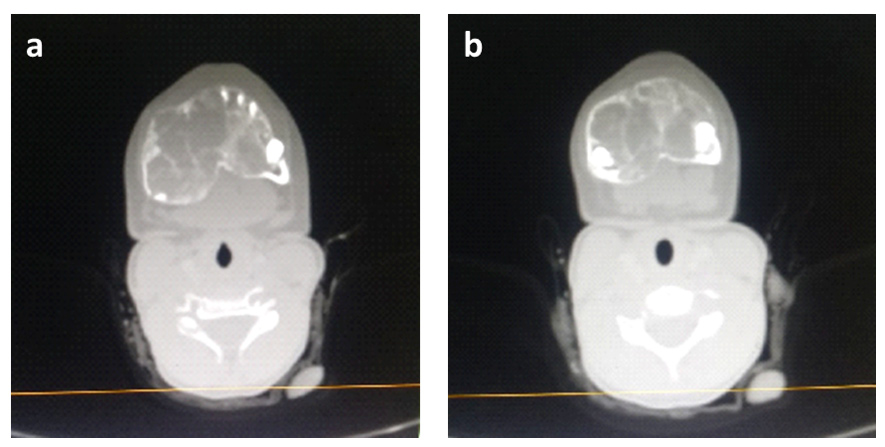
Computed tomography (CT) of the lesion. (
